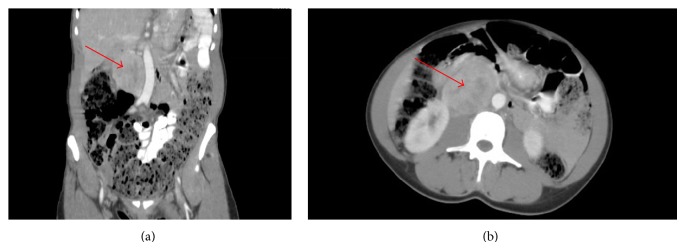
Paraganglioma (red arrow) visualized on computed tomography scan with IV contrast in coronal (a) and axial (b) planes without definitive evidence of local invasion.
